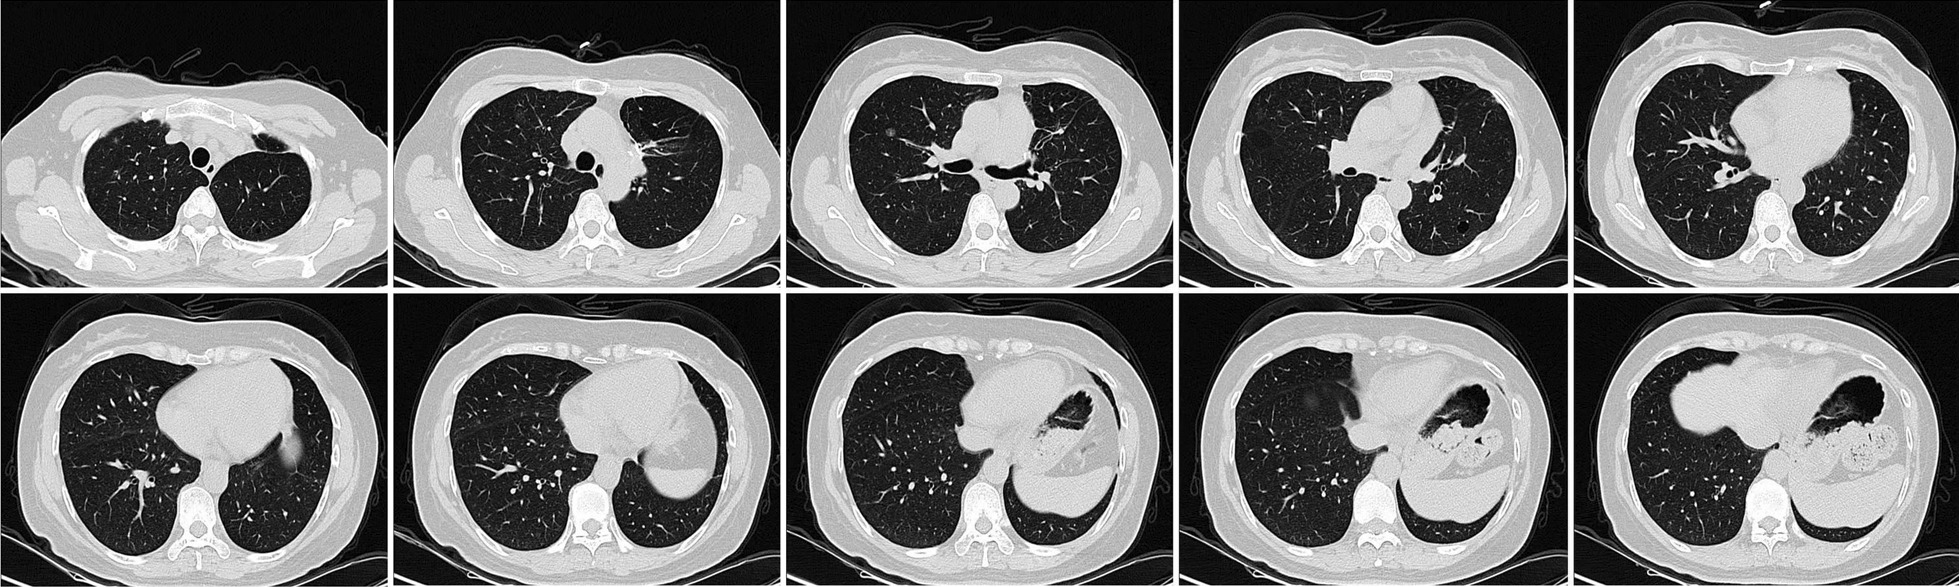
The postoperative computed tomography scanning. After 28 months of target therapy, most mGGNs in the right lung were completely absorbed, only one GGN in the right upper lung was radiologically same as before
